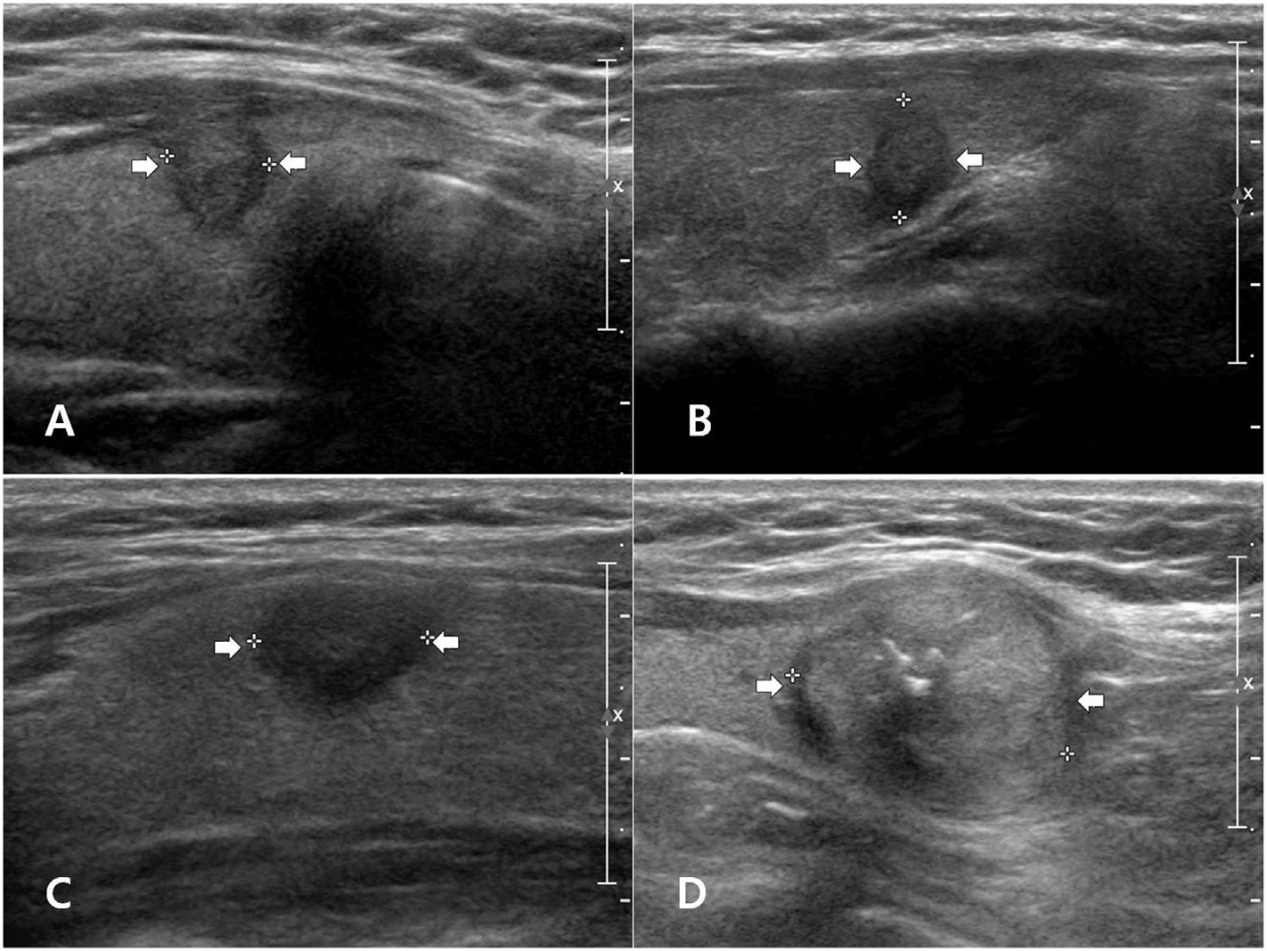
Examples of papillary thyroid carcinoma (PTC) subtypes with malignant ultrasonographic feature(s) on longitudinal gray-scale sonograms: classic PTC (A), follicular variant (B), tall cell variant (C), and oncocytic variant (D).
